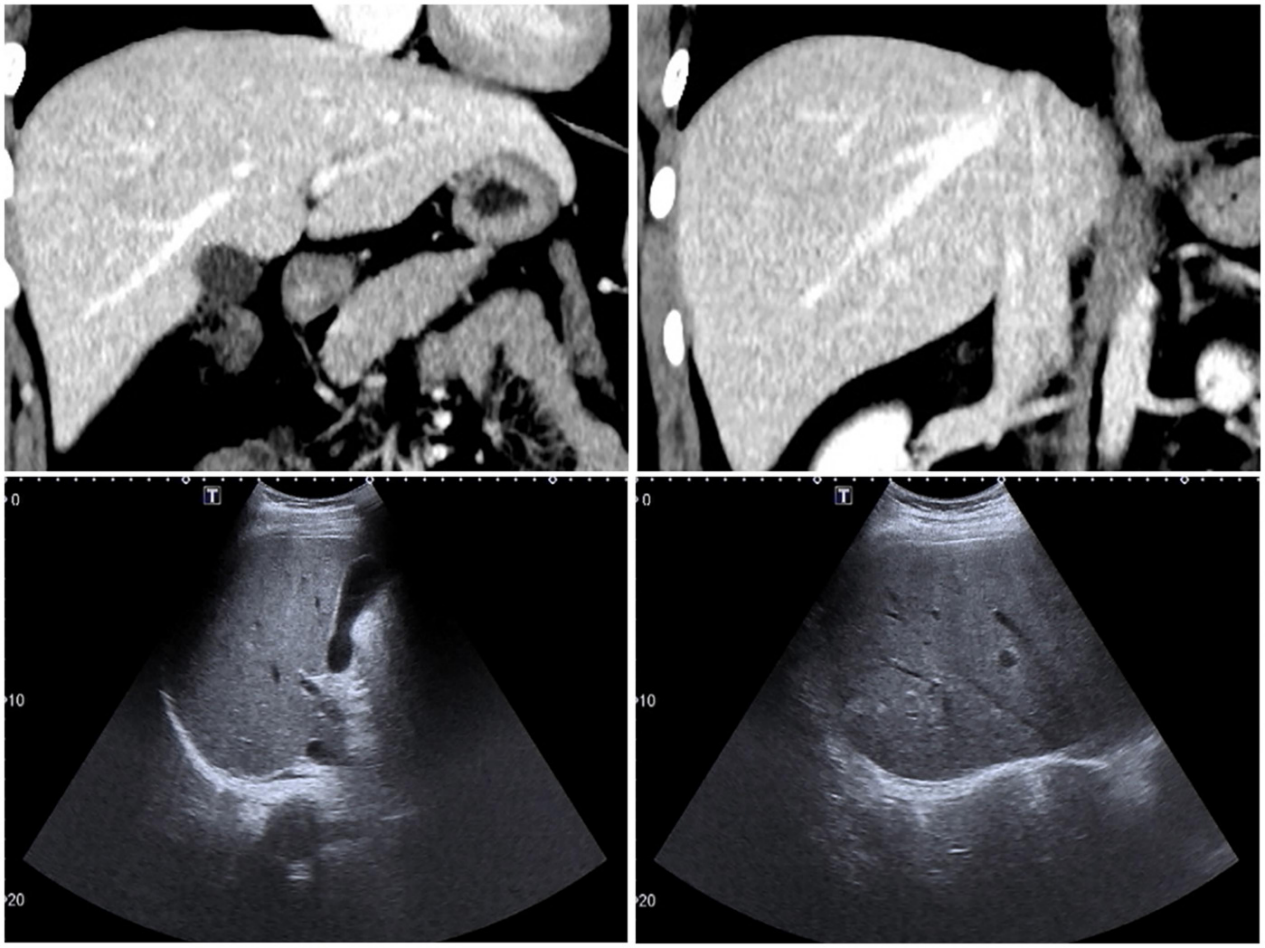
Ultrasound examination of liver.
